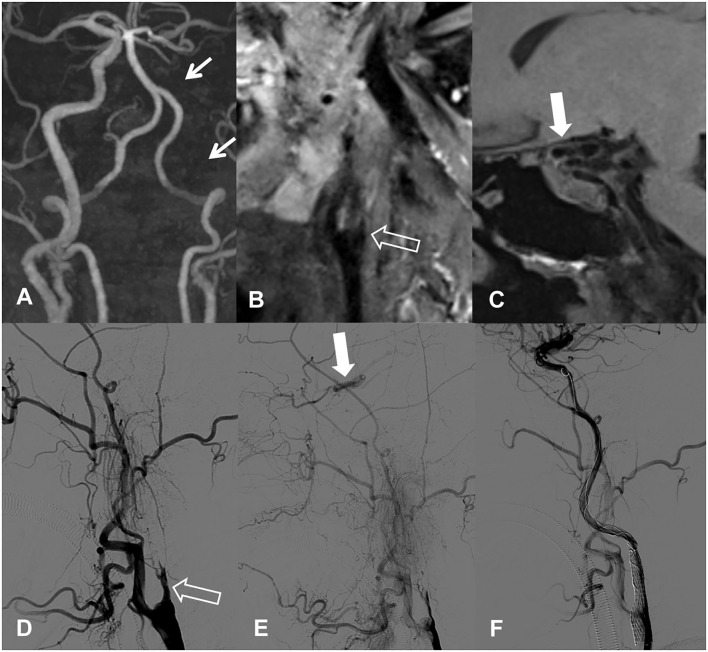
A 72-year-old female with right-sided limb weakness. (A) Long segmental occlusion of left internal carotid artery (ICA) is detected by TOF-MRA. (B,C) HR-MRI shows occlusion of proximal ICA with tapered stump and patent lumen of ophthalmic artery segment and above. This patient had scored as point 0 before recanalization. (D,E) Lateral images on DSA confirm the tapered stump condition and reversed flow from the ophthalmic artery. (F) Successful recanalization of the left ICA is achieved, followed by stent implantation.
